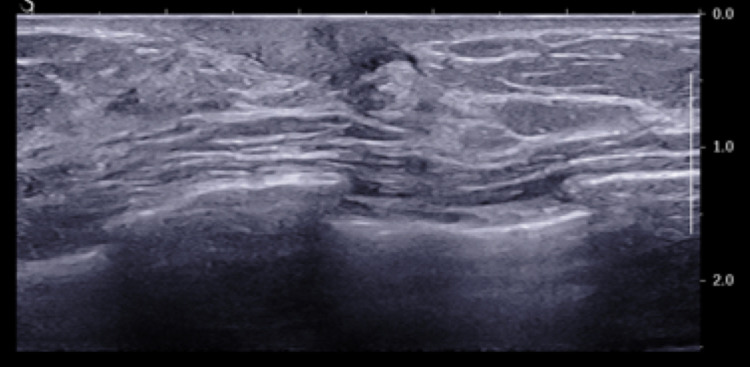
Representative static ultrasound image of the right subareolar breast demonstrating no suspicious mass.
